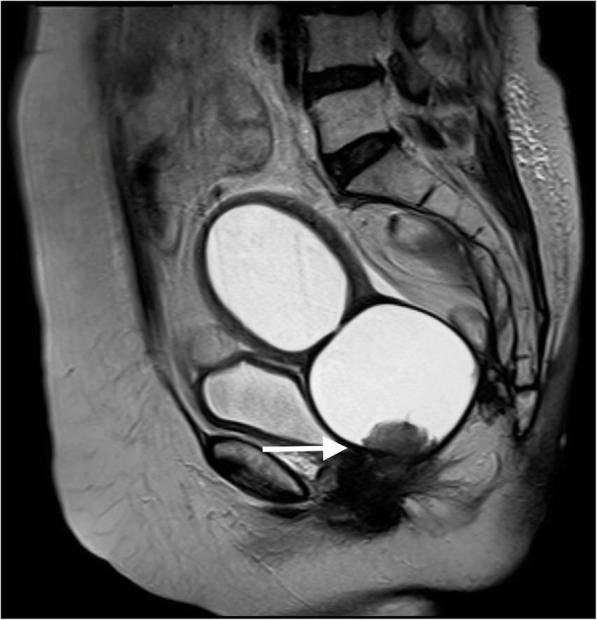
Sagittal T2-weighted image demonstrates increased T2 signal intensity representing water, filling endometrial and vaginal lumen secondary to obstruction of metastatic lesion (arrow) at the vaginal lumen distally
